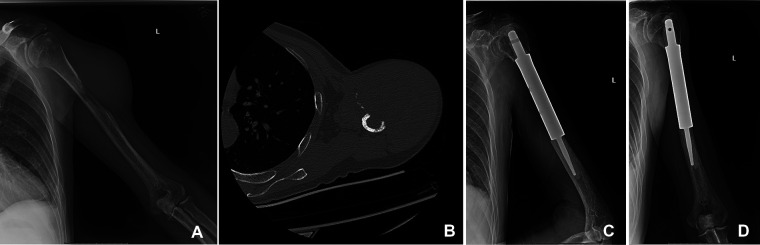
A 50-year-old man suffered from HCC with metastasis of the left humerus. The primary lesion of the liver was resected. (A,B) The bone metastasis in the middle part of the left humerus in x-ray and CT. (C,D) The patient underwent segmental tumor resection and artificial tumor prosthesis reconstruction.
